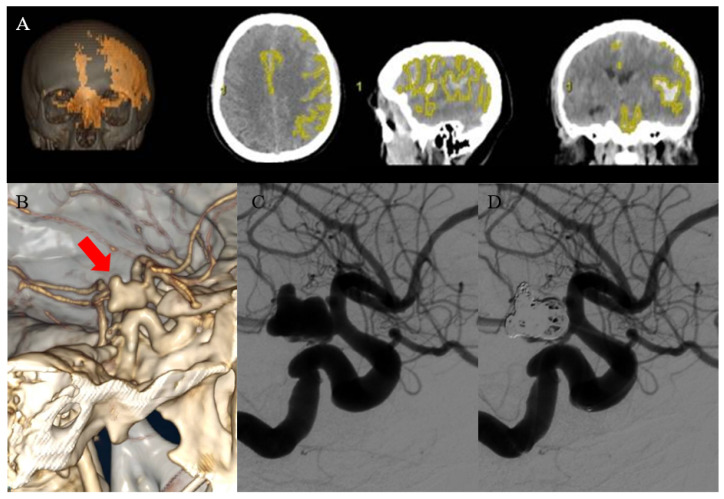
A case of a 63-year-old woman admitted with PG-aSAH (WFNS IV) due to the rupture of a posterior communicating artery aneurysm who presented a 6-month mRS of 2. (A) The CT scan on admission with semiautomatic hemorrhage volume evaluation in volume rendering (VR), axial, sagittal and coronal. (B) CT angiography demonstrating the presence of the ruptured posterior communicating artery aneurysm (arrow). DSA of the aneurysm before (C) and after (D) the endovascular treatment.
